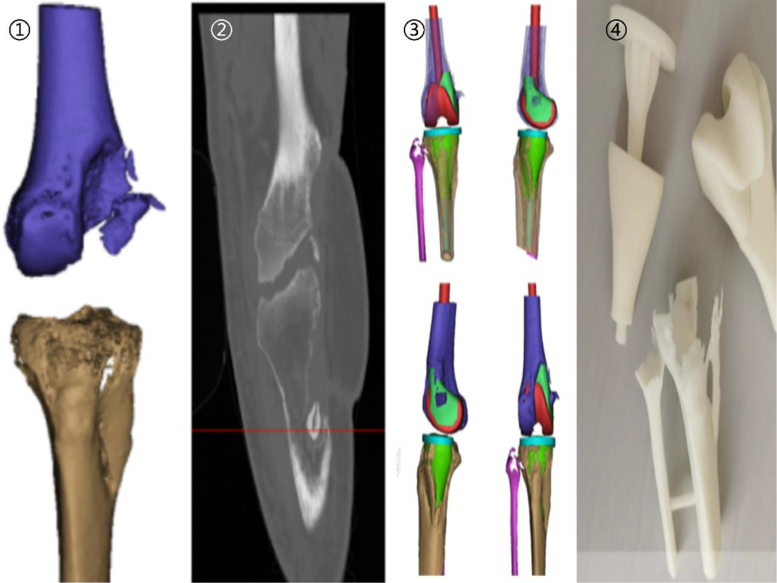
The patient underwent CT scanning and 3D reconstruction (Images 1 and 2), and 3D printing scans and models were created from the imaging data on the computer (Images 3 and 4). CT = computed tomography.
